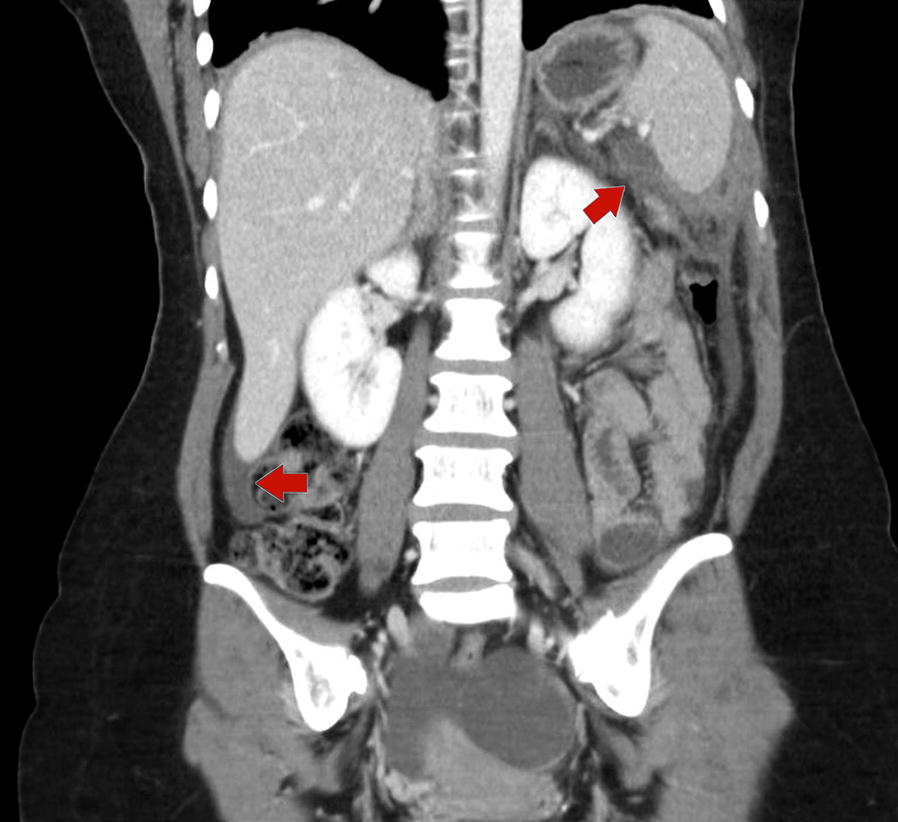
Computed tomography of the abdomen demonstrating omental thickening (arrows)
